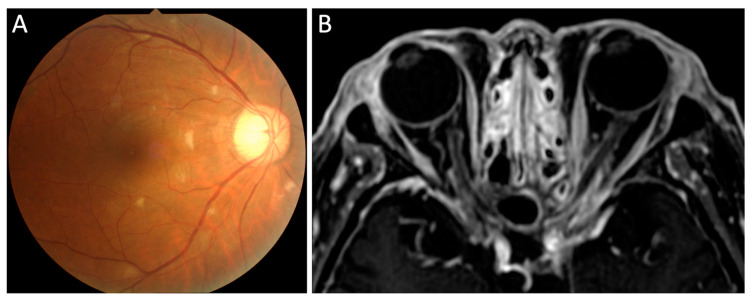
Retinopathy and optic perineuritis in patients with RPC. Fundus photograph shows cotton-wool spots in the retina (A). Gadolinium-enhanced T1-weighted MRI with fat suppression shows the “tram-track” enhancement of the left optic nerve sheath (B).
